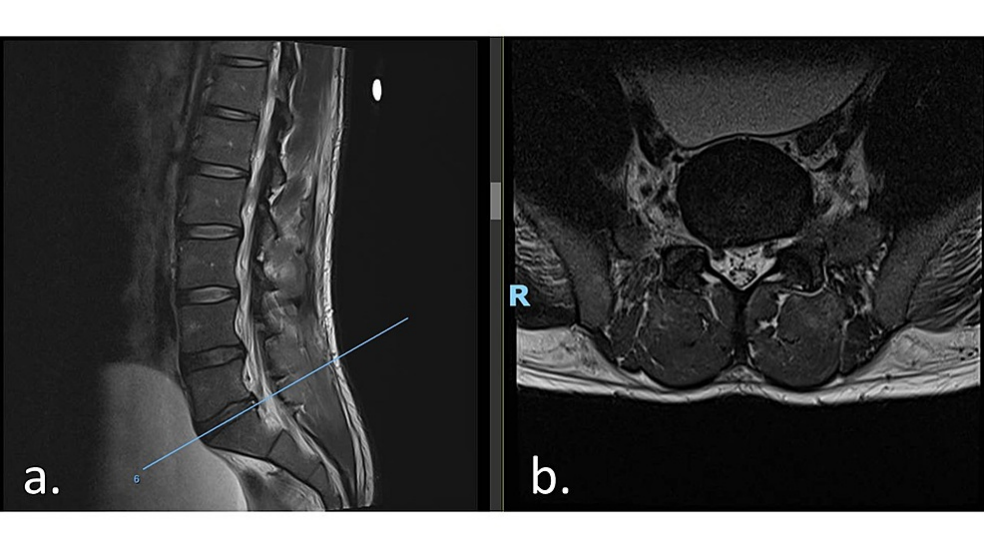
MRI lumbar spine showing L5/S1 diffuse disc bulge with a right paracentral annular fissure, mild right subarticular zone stenosis, with the disc contacting the right descending L5 nerve root (a) Sagittal cut. (b) Axial cut. MRI: magnetic resonance imaging, R: right.
